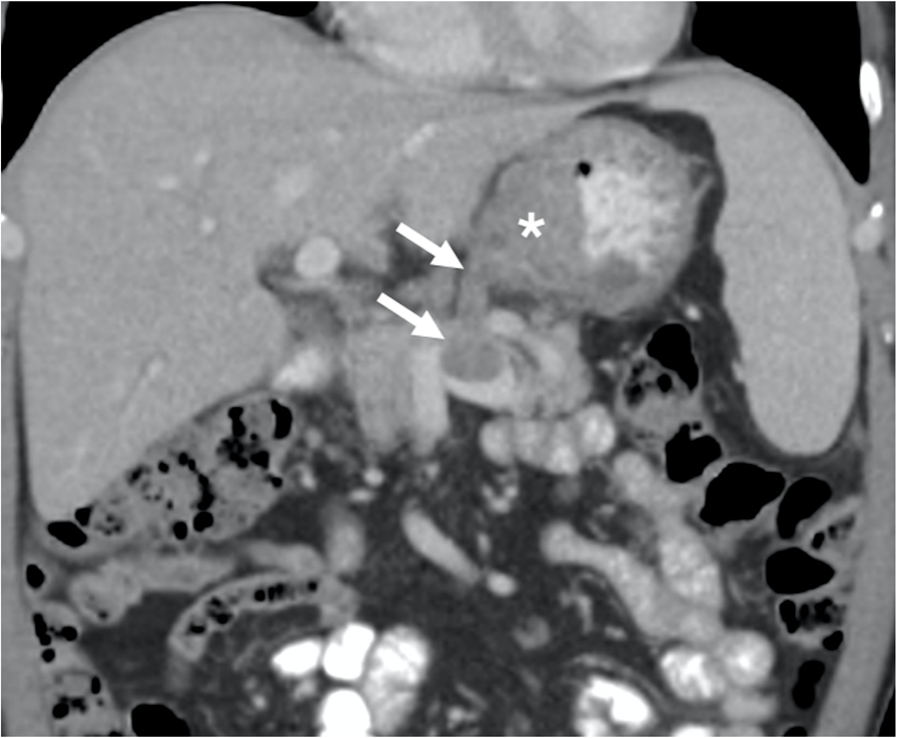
A 45-year-old male with newly diagnosed gastric cancer. Coronally reformatted postcontrast CT image shows the primary tumor in the gastric wall (asterisk) with tumor thrombus (arrows) extending into the splenic vein
